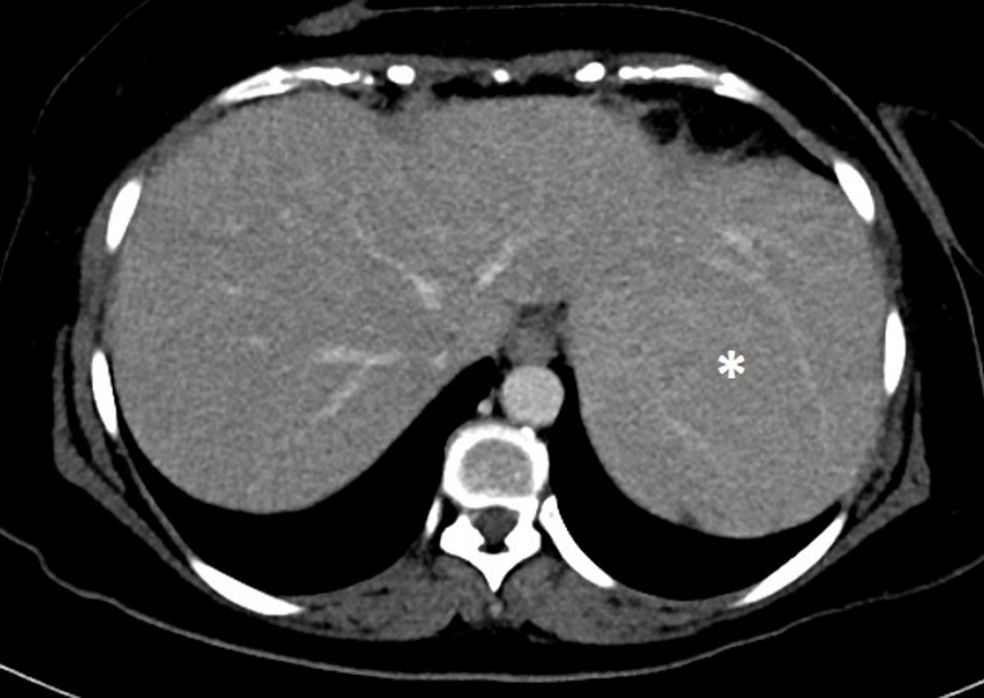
Selected axial image of contrast-enhanced CT mildly enlarged liver mainly the left lobe, which is extending to the lateral side of the left upper quadrant of the abdominal cavity. Left lobe of the liver (*).
